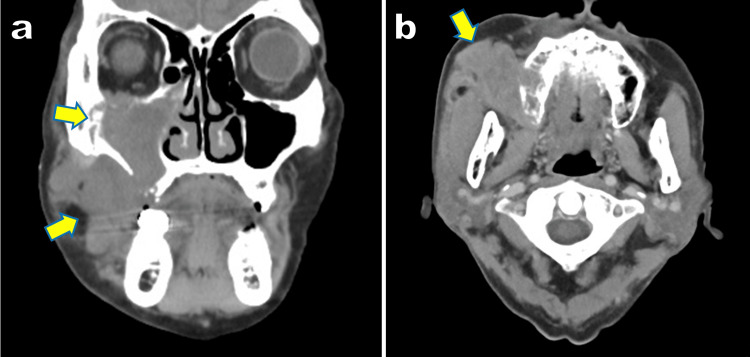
CT images. (a) Coronal section. (b) Axial section. A soft-tissue mass extending from the right maxillary sinus to the subcutaneous region of the right cheek and orbital floor. Destruction of the sinus wall and alveolar bone was also observed. The yellow arrows indicate the position of the soft-tissue mass.
